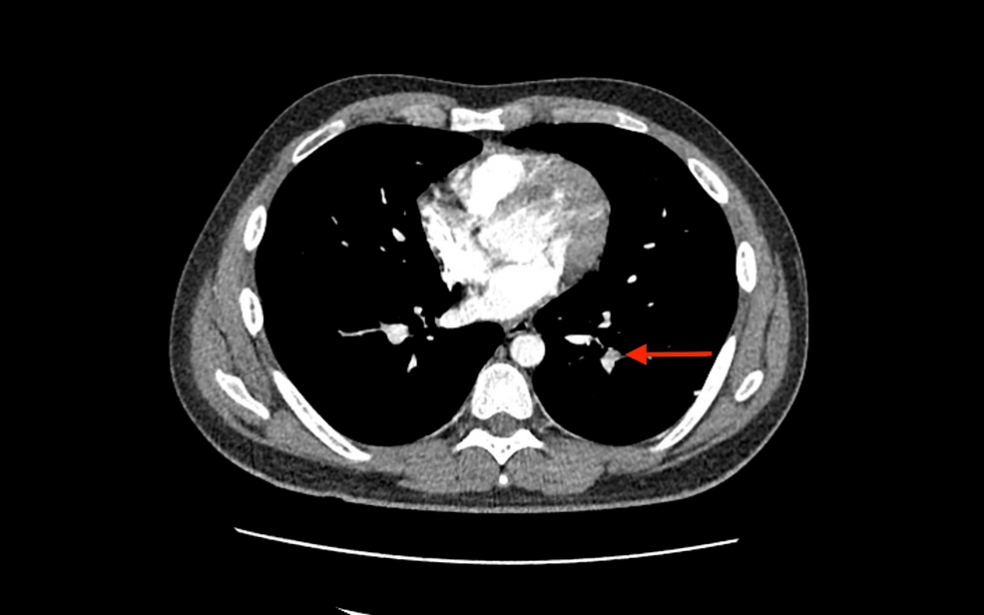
CT pulmonary angiogram demonstrating left lower lobe segmental filling defect (red arrow)
